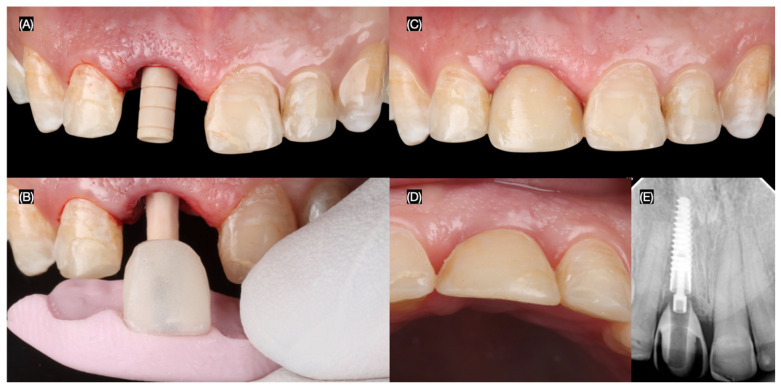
Implant provisional restoration. (A) Interim implant post; (B) placement of the interim crown with putty index, interim crown, (C) frontal, and (D) incisal view; and (E) radiograph.
